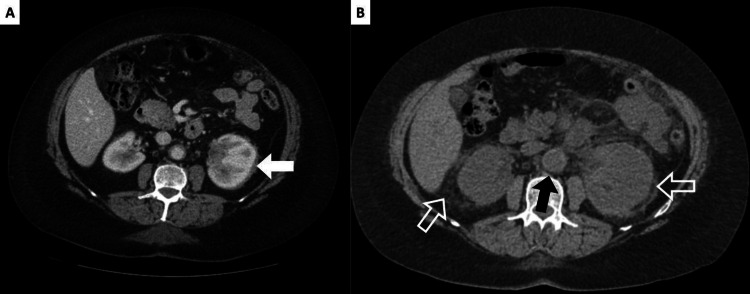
Axial CT scan of the abdomen (A) Initial axial CT scan of the abdomen demonstrating perinephric stranding with "hairy kidney" features (solid white arrow). (B) Axial CT scan of the abdomen at the one-year follow-up demonstrating periaortic thickening (solid black arrow) and expanded bilateral perinephric stranding and thickening (hollow white arrows) CT: computed tomography
